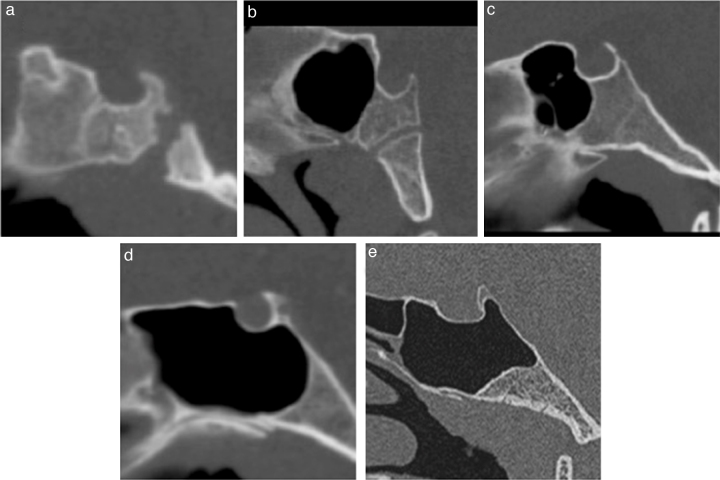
Sinus classification. Midsagittal reformatted images obtained from CT scans. (a–e) Types 1 through 5 sphenoid sinuses, respectively.
